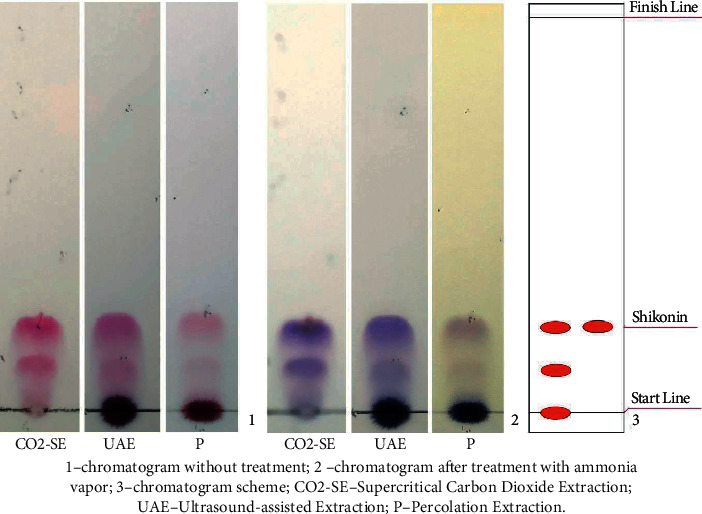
Thin-layer chromatogram of different extracts from the roots of *O. gmelinii*. (1) chromatogram without treatment; (2) chromatogram after treatment with ammonia vapour; (3) chromatogram scheme; CO2-SE: supercritical carbon dioxide extraction; UAE: ultrasound-assisted extraction; P: percolation extraction.
